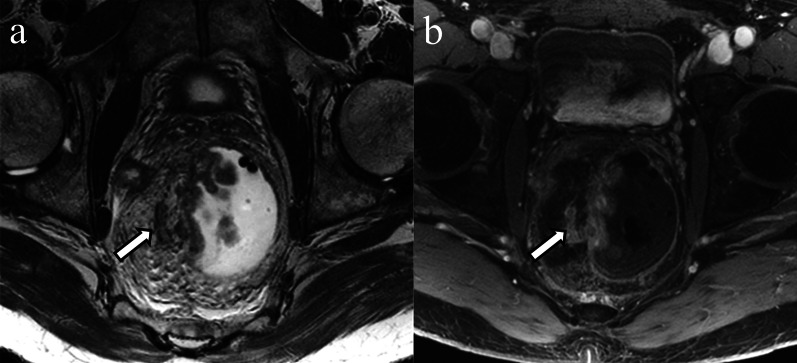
Extramural invasion on contrast-enhanced T1-weighted image. A 56-year-old male with rectal adenocarcinoma. The dilated vessel which is in continuity with the tumor has an irregular margin and contains intermediate signal intensity rather than flow void on the T2-weighted image (a arrow). The tumor in the vessel enhances on the contrast-enhanced T1-weighted image (b arrow)
